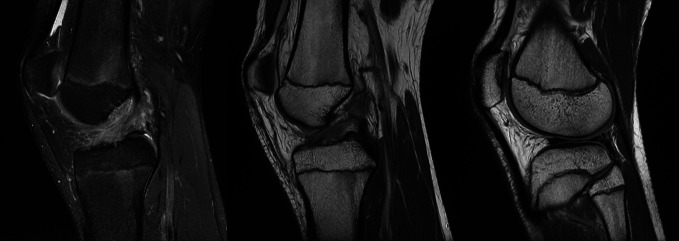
Sagittal T1-weighted and STIR-weighted MRIs showing the knee of a 15-year-old boy, with a complete anterior cruciate ligament rupture and associated meniscal tear.
